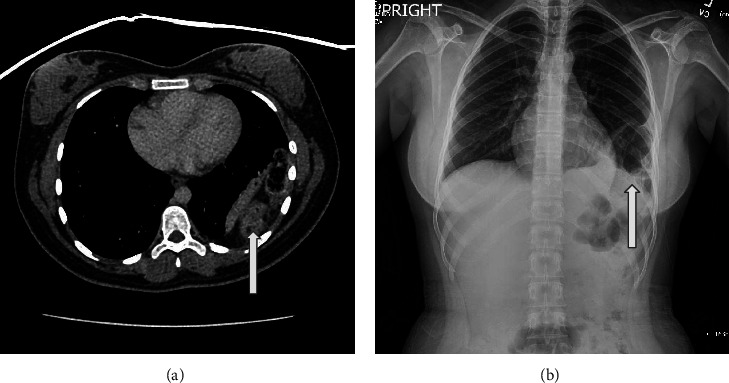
Imaging studies depicting bowel in the left lung field with defects in the left hemidiaphragm (arrows). (a) Chest CT. (b) Chest radiograph.
